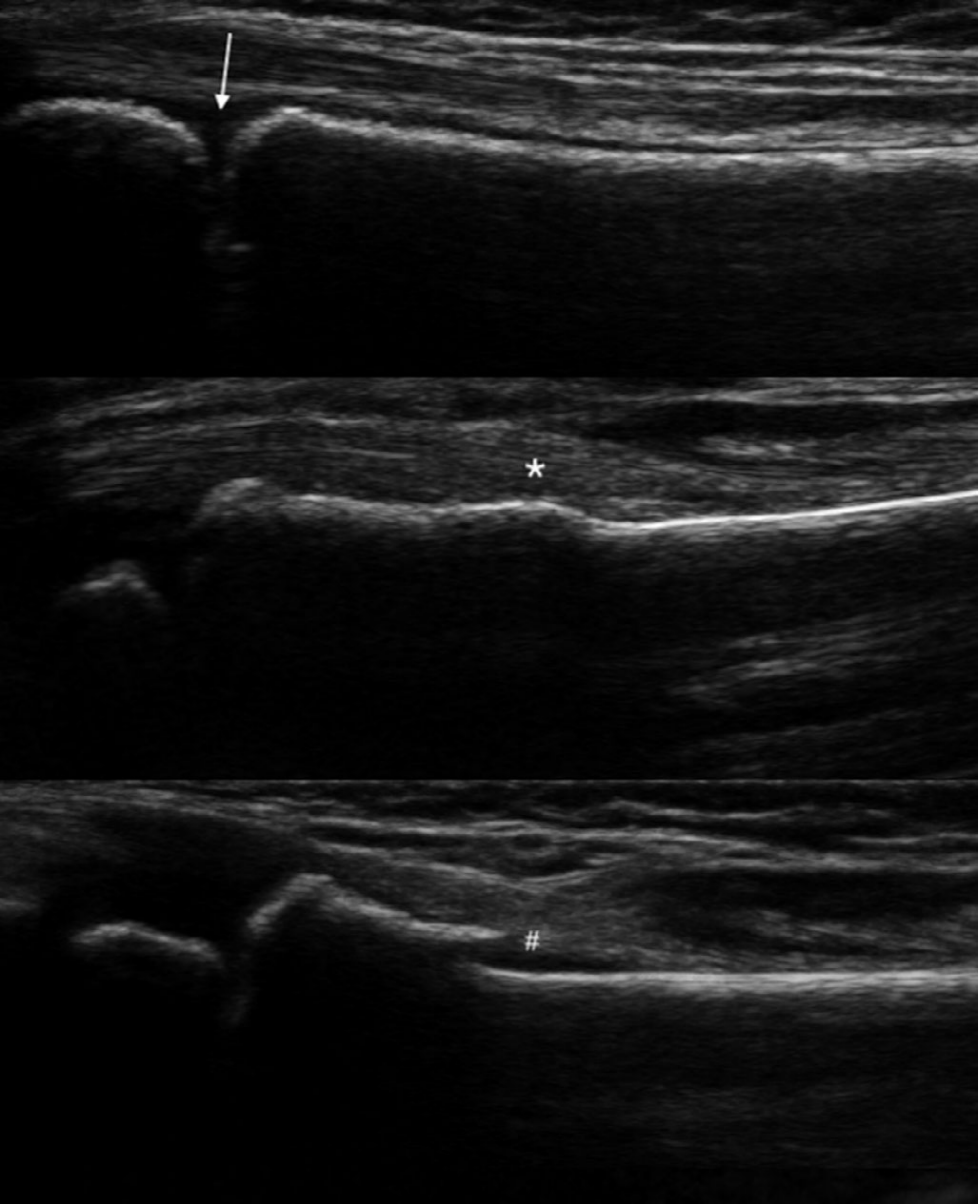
Distal radius ultrasound images demonstrating the different forearm injury categories: no fracture (arrow pointing to physis), buckle fracture (*) and cortical breach fracture (#).
